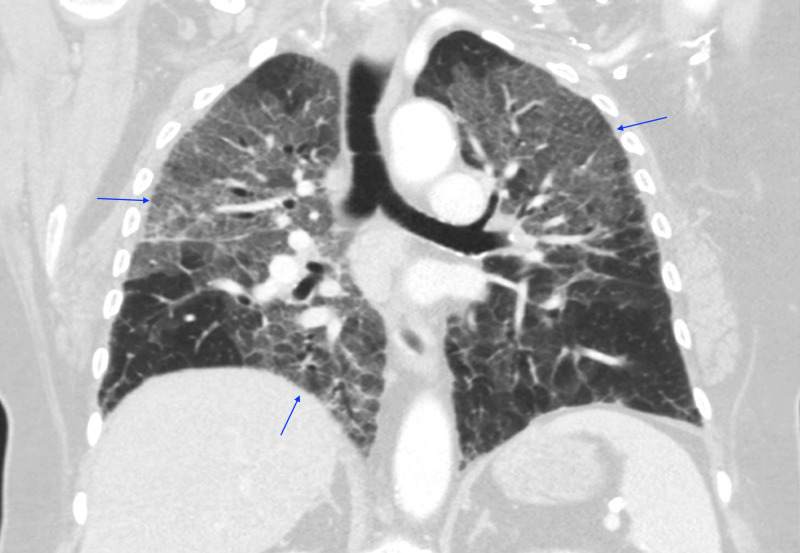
CT Chest (Coronal View) Multifocal ground-glass opacities predominately affecting the right lung in addition to the worsening of the left apical lobe comparing to the previous study.
